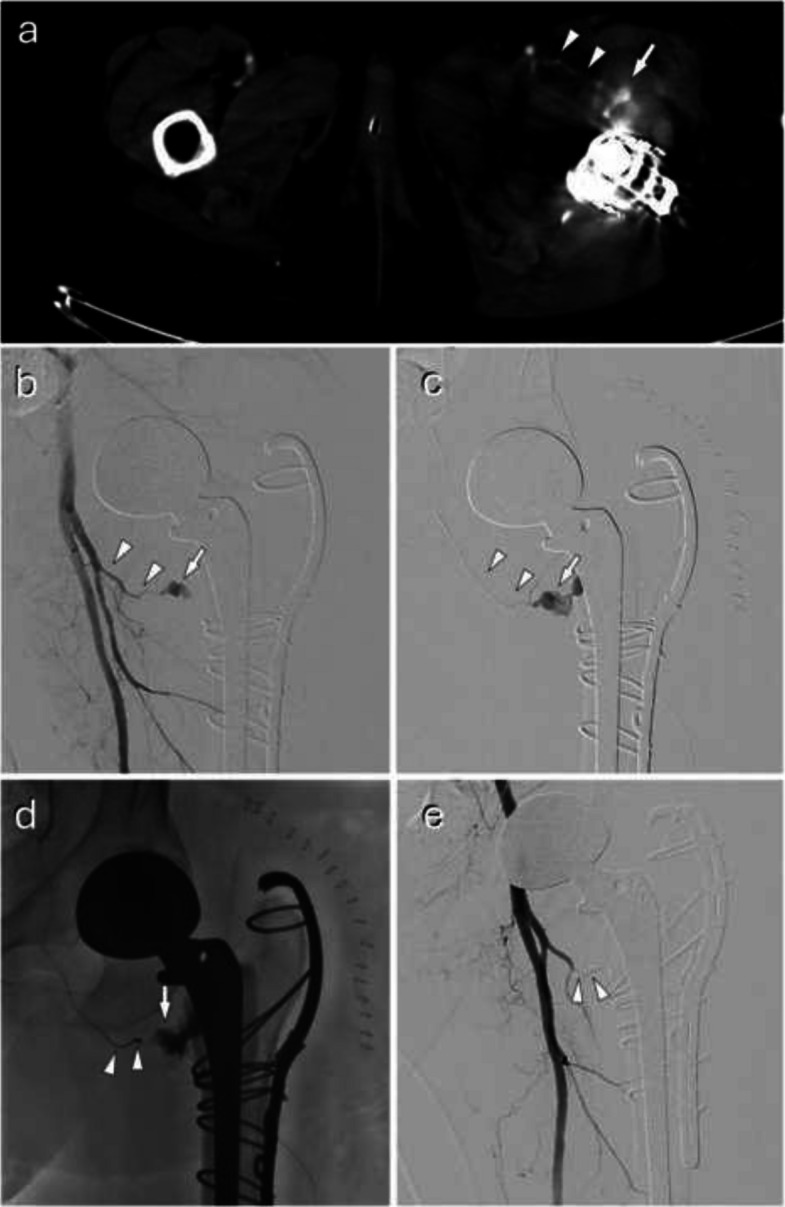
A postoperative active bleeding managed by coiling. A 58-year-old patient undergoing revision of a left total hip arthroplasty with cerclage. Postoperatively, the patient developed a thigh hematoma with acute anemia but remained hemodynamically stable. a Contrast-enhanced CT identified active bleeding (arrow) from a proximal branch of the profunda femoris artery: the ascending branch of the lateral circumflex femoral artery (arrowheads). He was managed by interventional radiology after multidisciplinary discussion. The procedure was performed under sedation and local anesthesia. Using a cross-over approach, a 6 F catheter was used for angiography (b), and superselective catheterization with a 2.7F microcatheter (c), which confirmed CT findings (same annotations). d Embolization of the bleeding branch was performed using multiple coils (arrowheads), just proximal to the site of contrast extravasation (arrow). e Post-embolization angiography shows complete occlusion of the ascending branch of the lateral circumflex femoral artery by coils (arrowheads), and contrast extravasation resolution
